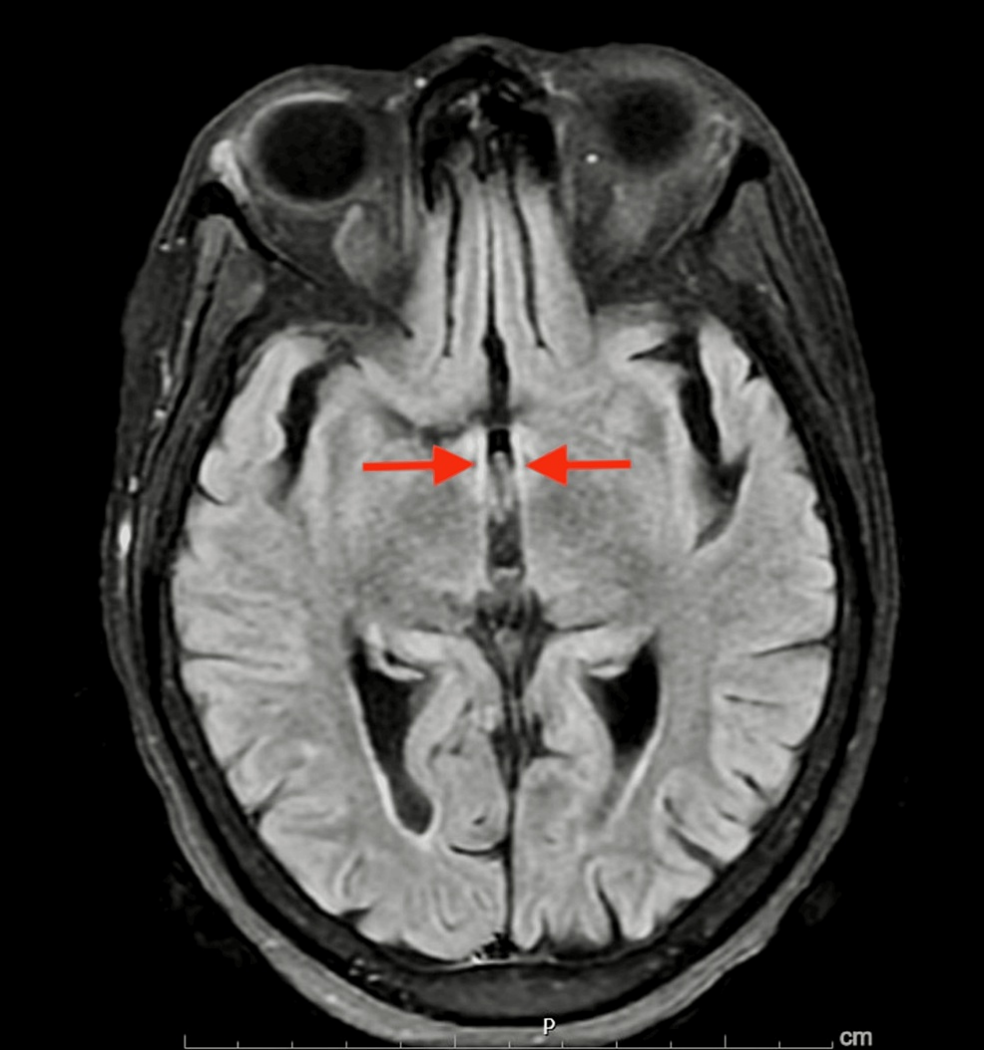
Non-contrast MRI, axial view showing increased T2 FLAIR signal affecting the bilateral mammillothalamic tracts (red arrows). FLAIR: Fluid-attenuated inversion recovery
